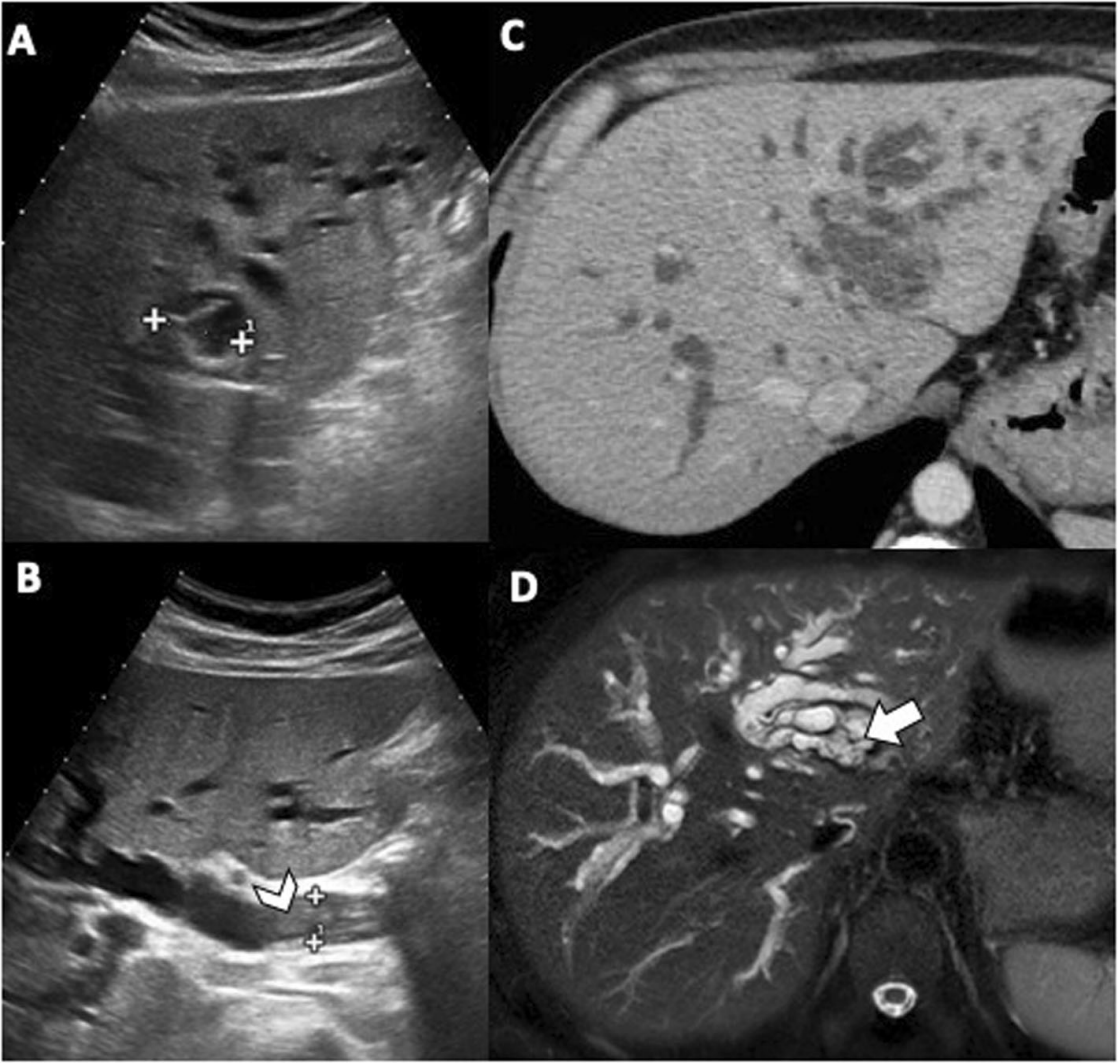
A 48-year-old man with hydatid liver cyst complicated with communicating rupture. a, b US images show a focal anechoic liver lesion with internal septa and hyperechoic peripheral rim, with some posterior shadows, diffuse biliary dilatation and endoluminal hyperechoic foci (arrowhead) into the common bile duct with no posterior acoustic shadows. Axial CT scan in the portal venous phase (c) and MR image on T2-weighted sequence (d) confirm the presence of a liver cyst and diffuse biliary dilatation, with material into the biliary tree (arrow). These features are consistent with progressive migration of the hydatid sand and membranes from the hilar confluence to the common bile duct
